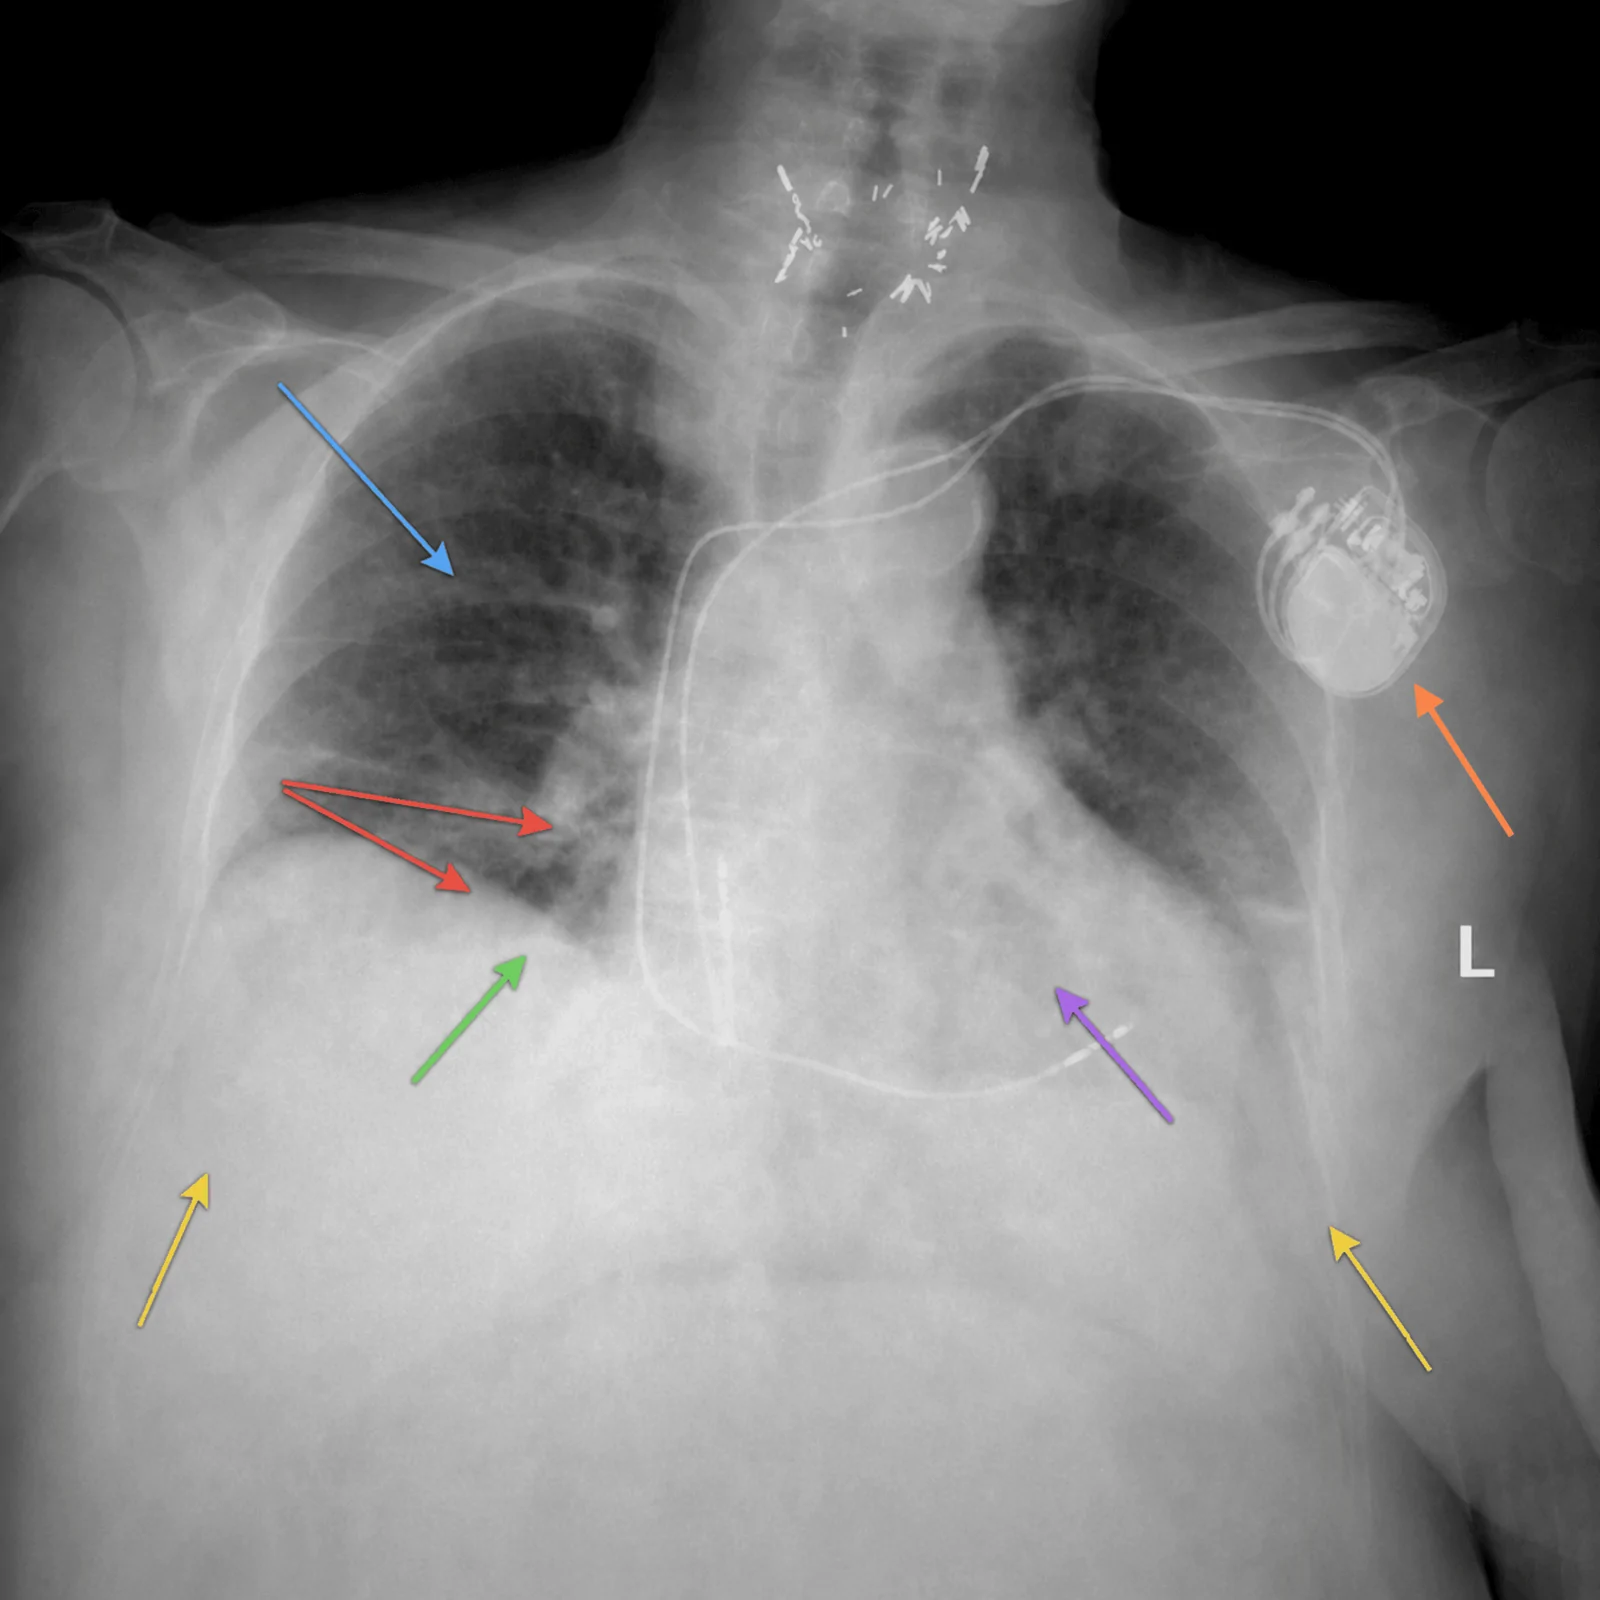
Chest X-ray X-ray of the chest showing mild pulmonary vascular congestion (blue arrow), bibasilar infiltrates (red arrow) and atelectasis (green arrow) with small bilateral pleural effusions (yellow arrow), which were questionable for pneumonia, and cardiomegaly (purple arrow) with a left-sided pacemaker (orange arrow).
